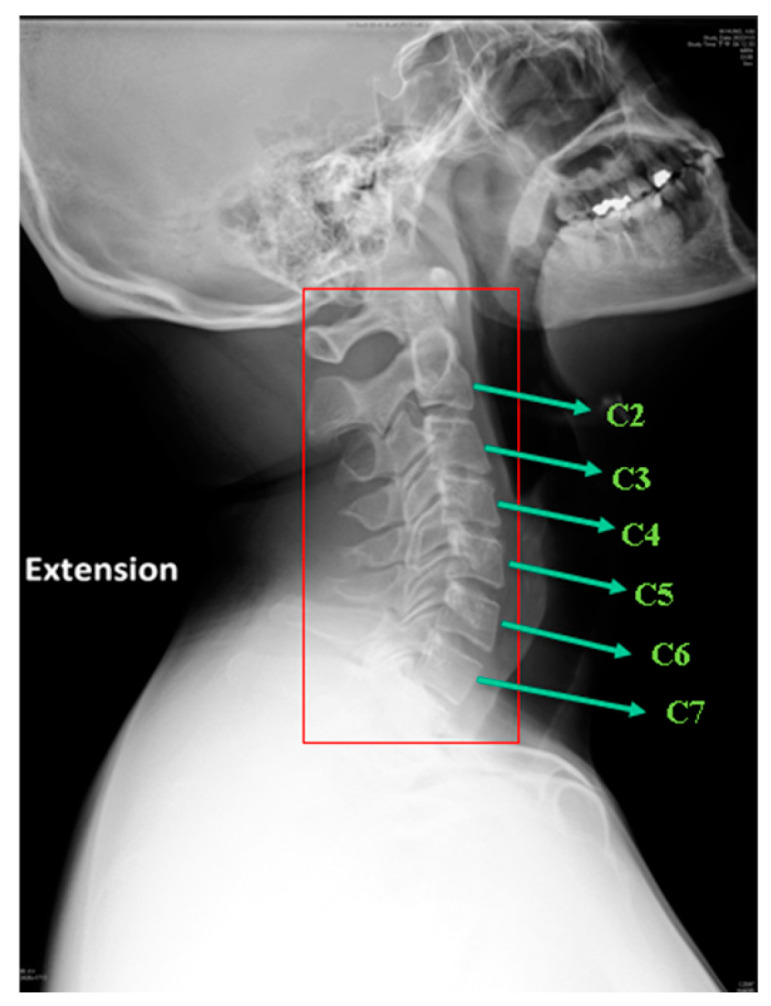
The cervical spine radiography with cervical vertebrae labels.
